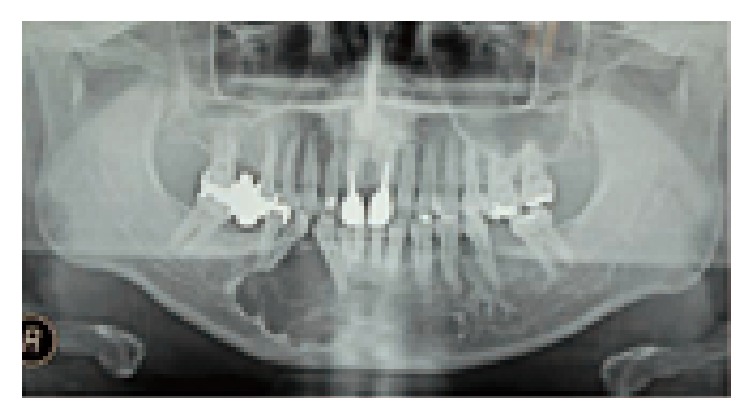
A panoramic radiograph showing a well-defined large radiolucent lesion in the right mandible.
